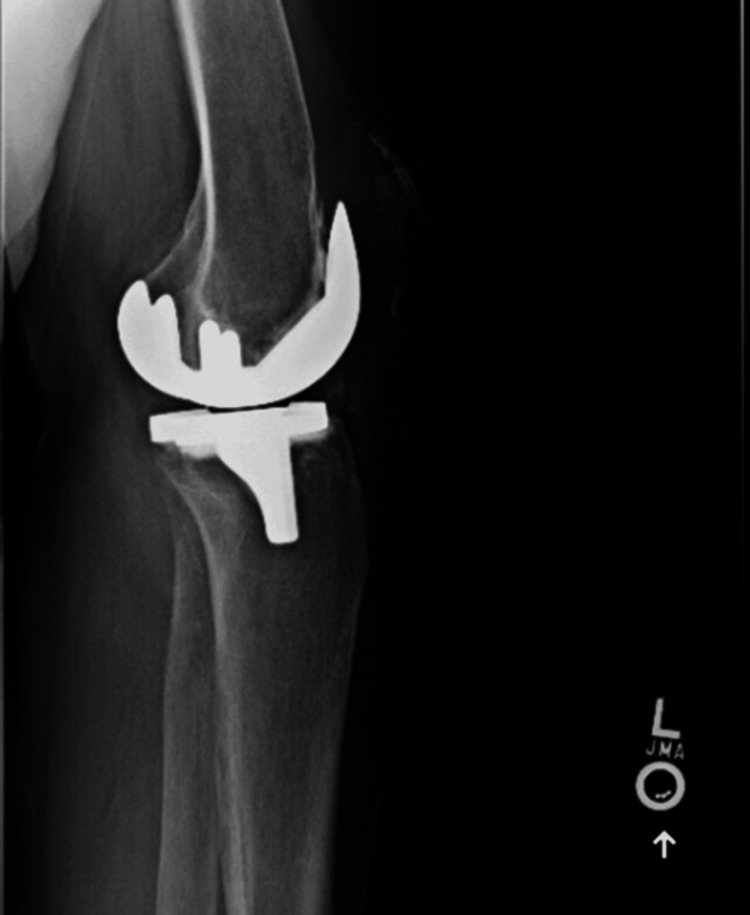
Preoperative lateral radiograph of the left knee demonstrating resorption of the left patella
